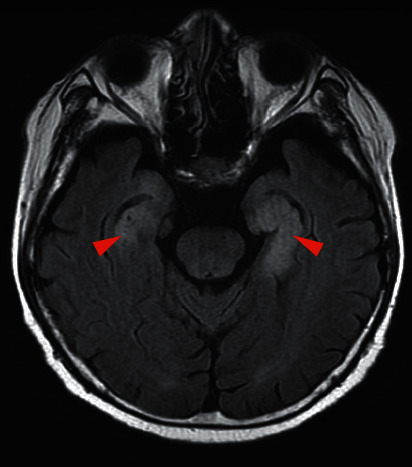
FLAIR image of brain MRI after development of neurological symptoms reveals high-intensity area in bilateral temporal lobes (red arrowheads).
